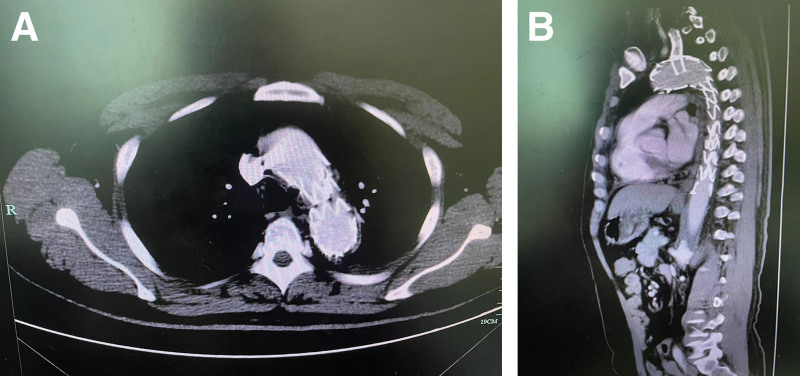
CTA image after endovascular isolation of aortic dissection by covered stent within 1 month. (A) transverse section and (B) sagittal section.
